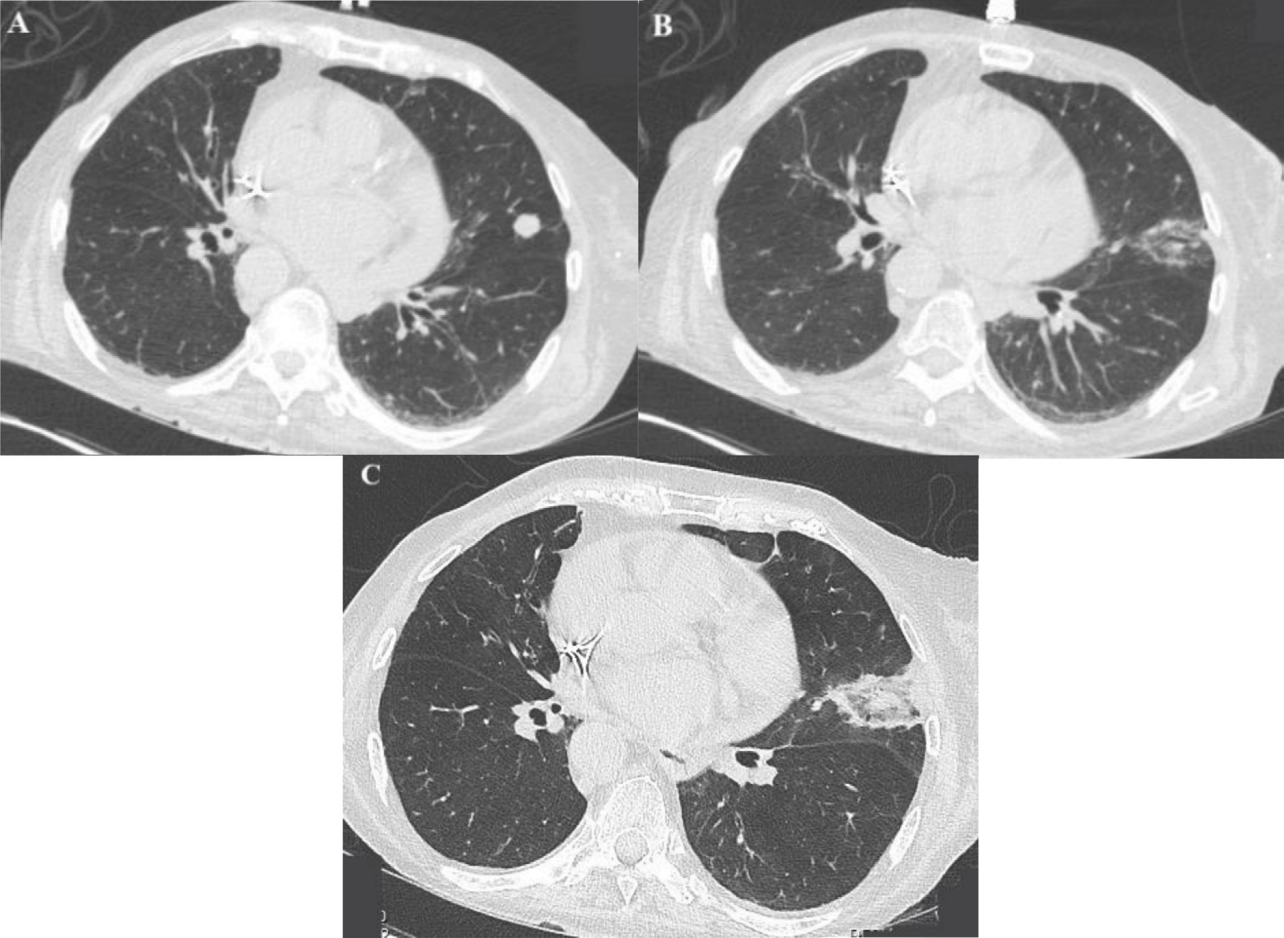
Axial CT images of the pulmonary carcinoid tumor during the ablation procedure before and after (A and B), and on post‑procedural day 1 (C), demonstrating ablation zone and absence of immediate complications.
